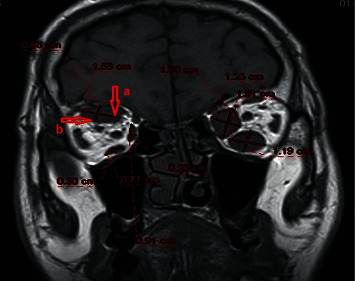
Measurement technique of muscle diameters on MRI. Arrow a shows long diameter and arrow b shows short diameter.
